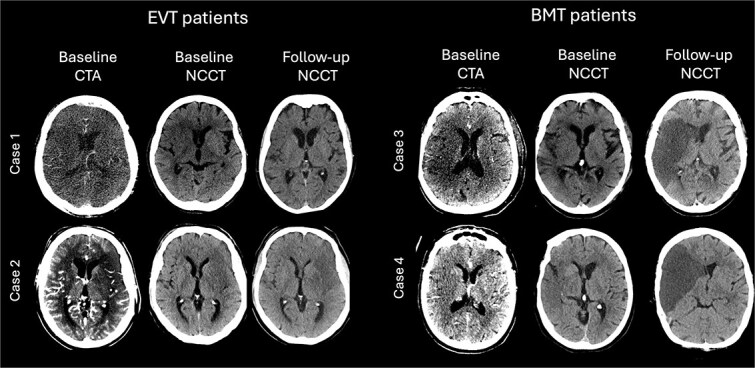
Patient examples. Four patients (case 1/2: EVT, case 3/4: BMT) showing baseline imaging and follow-up CT. In accordance with the main results of this study, follow-up imaging shows a significant reduction in oedema formation after EVT. Case 1/2: ΔNWU of 2.0%/1.5%; case 3/4: ΔNWU: 20.4%/19.6%.
